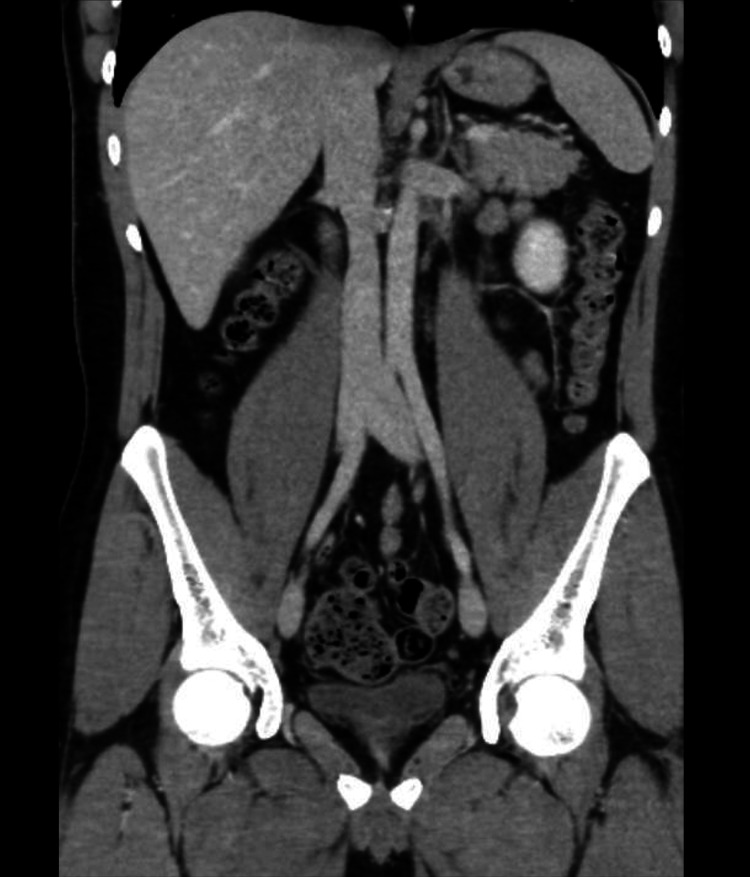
Coronal section CT scan of the abdomen after four cycles of chemotherapy The image demonstrated a patent IVC with no evidence of thrombus, along with resolution of para-aortic lymph node enlargement IVC: inferior vena cava; CT: computed tomography
